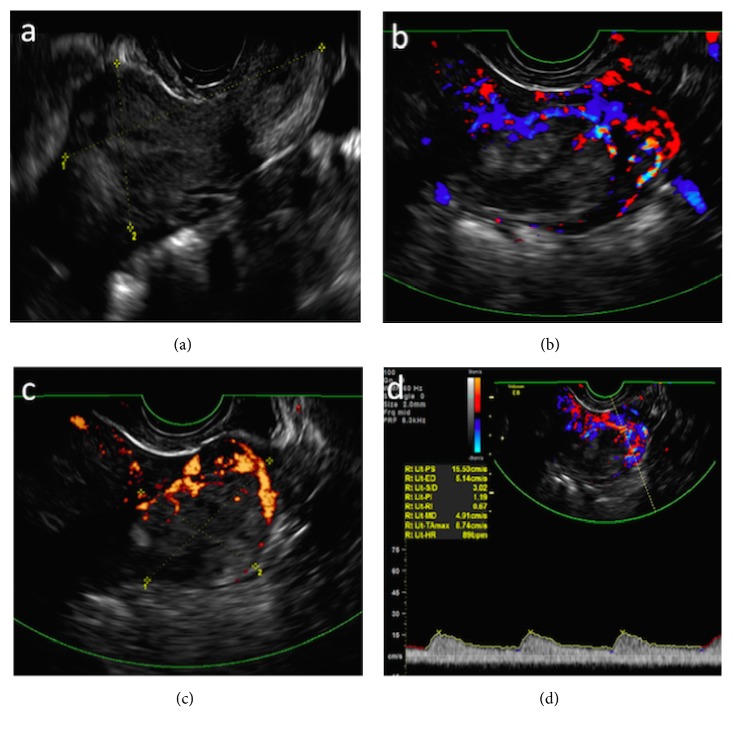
(a) Transvaginal sonographic sagittal section of the uterus revealing no signs of intrauterine pregnancy. (b) Color Doppler and (c) power Doppler transvaginal sonographic transverse sections of the left paraovarian tumor with strong peripheral vascularization. (d) Pulsed Doppler analysis of tumor vascularization, showing a pattern of low resistance.
